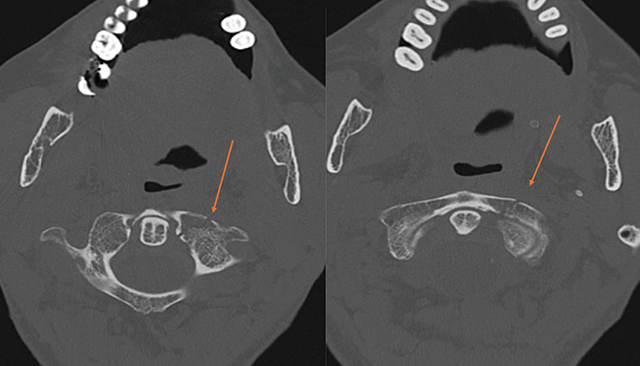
Axial CT scan (bone window) showing a non-displaced fracture of the left anterior arch of the C1 vertebra.
